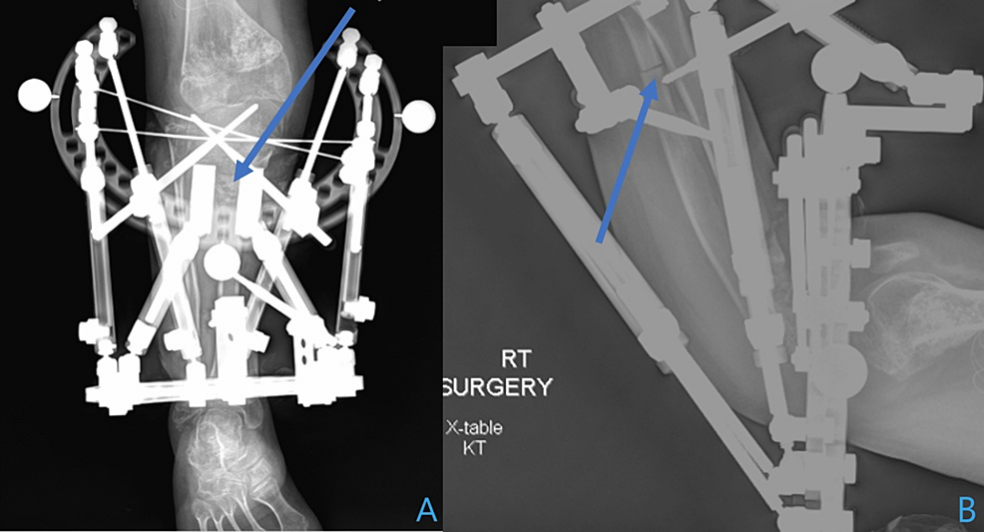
Immediate post-operative radiography Anteroposterior (A) and lateral (B) of the right leg immediately post-operatively demonstrating the circulator external fixator with a 155 5/8 ring proximally, a 105 full ring distally, six struts, and two z-plates used to offload the proximal struts. The proximal tibial and fibular osteotomy and distal fibular osteotomy fixation are pointed out (blue arrows). The external fixator is fixated to the proximal tibia and the distal tibia/fibula syndesmosis.
